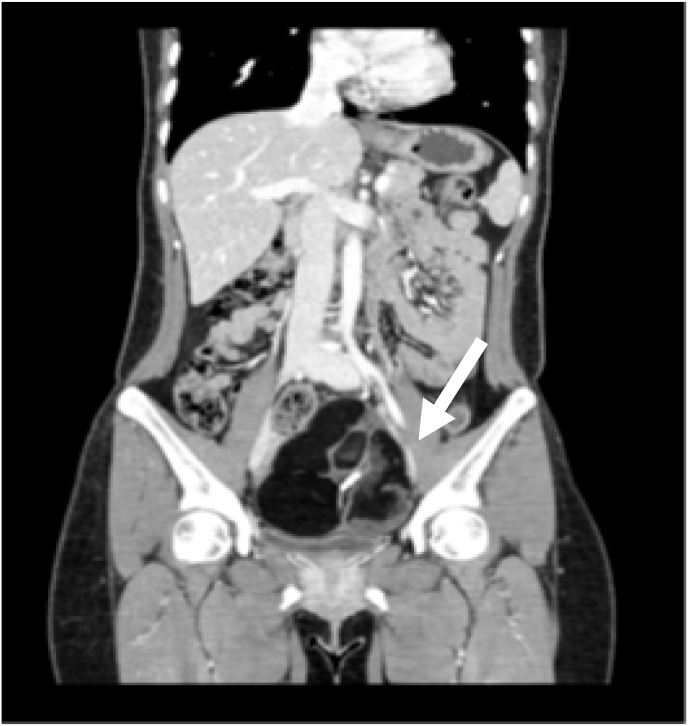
A computed tomography scan showing a well-defined multi-septated fatty mass with dense internal calcification and mild septal enhancement in the left ovarian fossa (white arrow).
